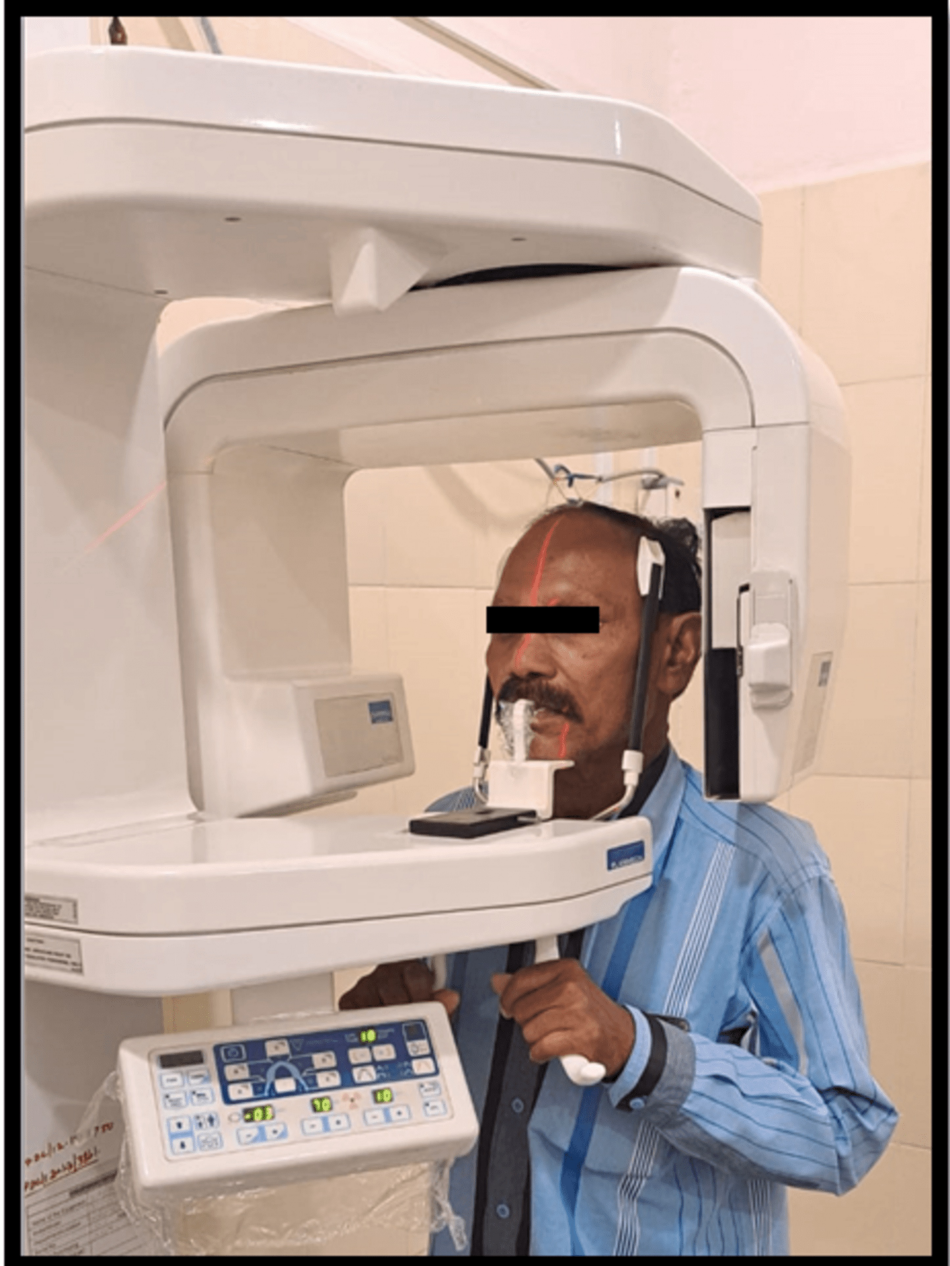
Position of head for shooting OPG OPG: Orthopantomogram
